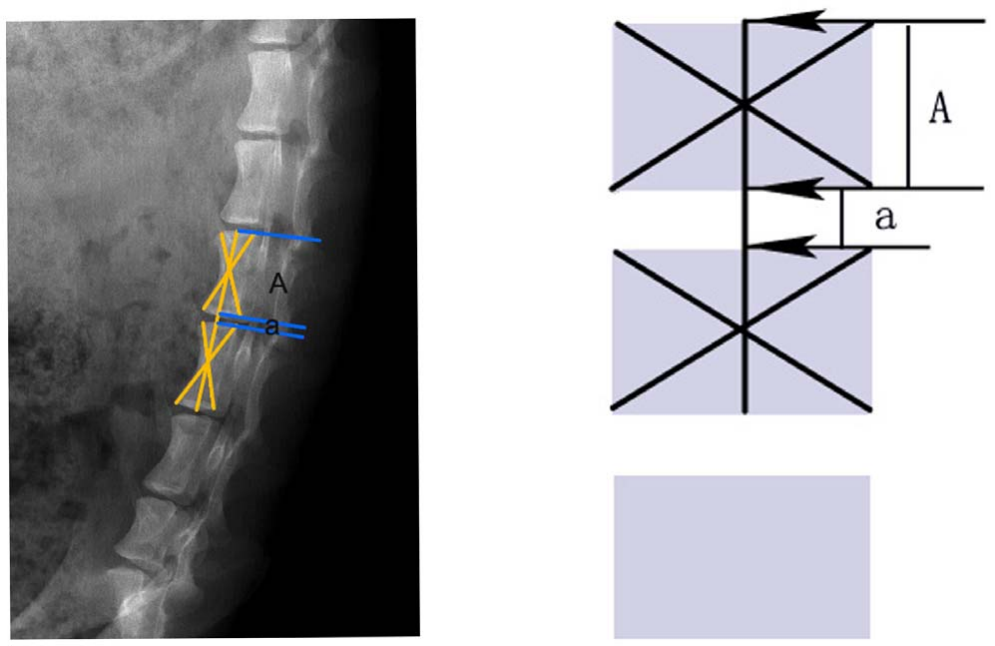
Radiographic measurement of DHI. Heights of the vertebral body and disc were measured using the middle vertebral line. DHI = a/A.
